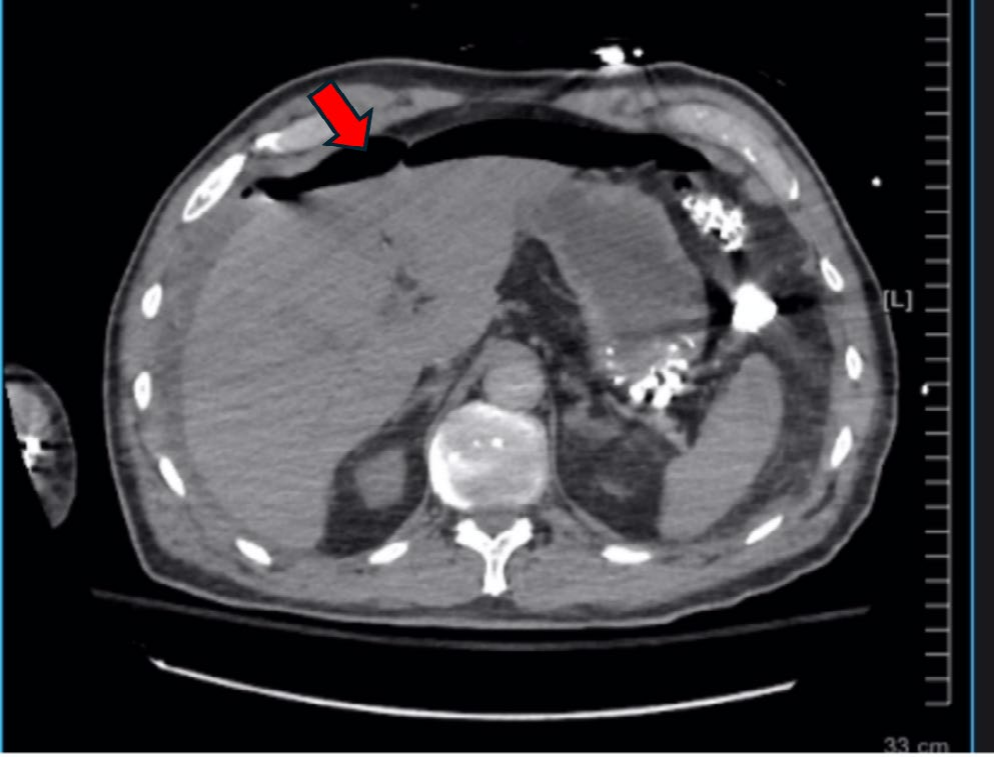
CT angiogram of the chest. CT scan shows extensive free air under the hemidiaphragm (red arrow). There is also free fluid in the abdomen.
